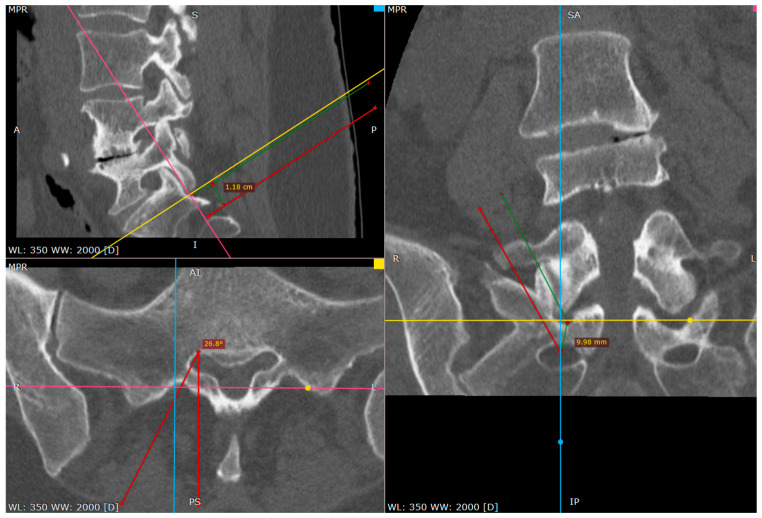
Distance between inferior facet surface and dorsal foramina (CT tri-plane reconstruction).
